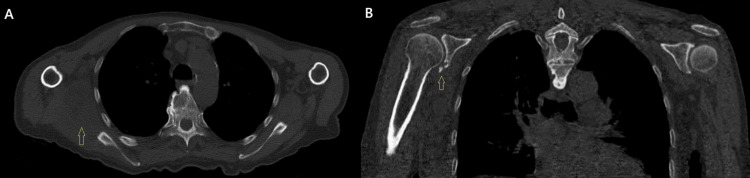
CT one month after the injury. (A) Transverse view depicting the significantly reduced hematoma (indicated by the yellow arrow). The lateral displacement of the right scapula had resolved. (B) Coronal view exhibiting the fracture of the right scapular glenoid.
